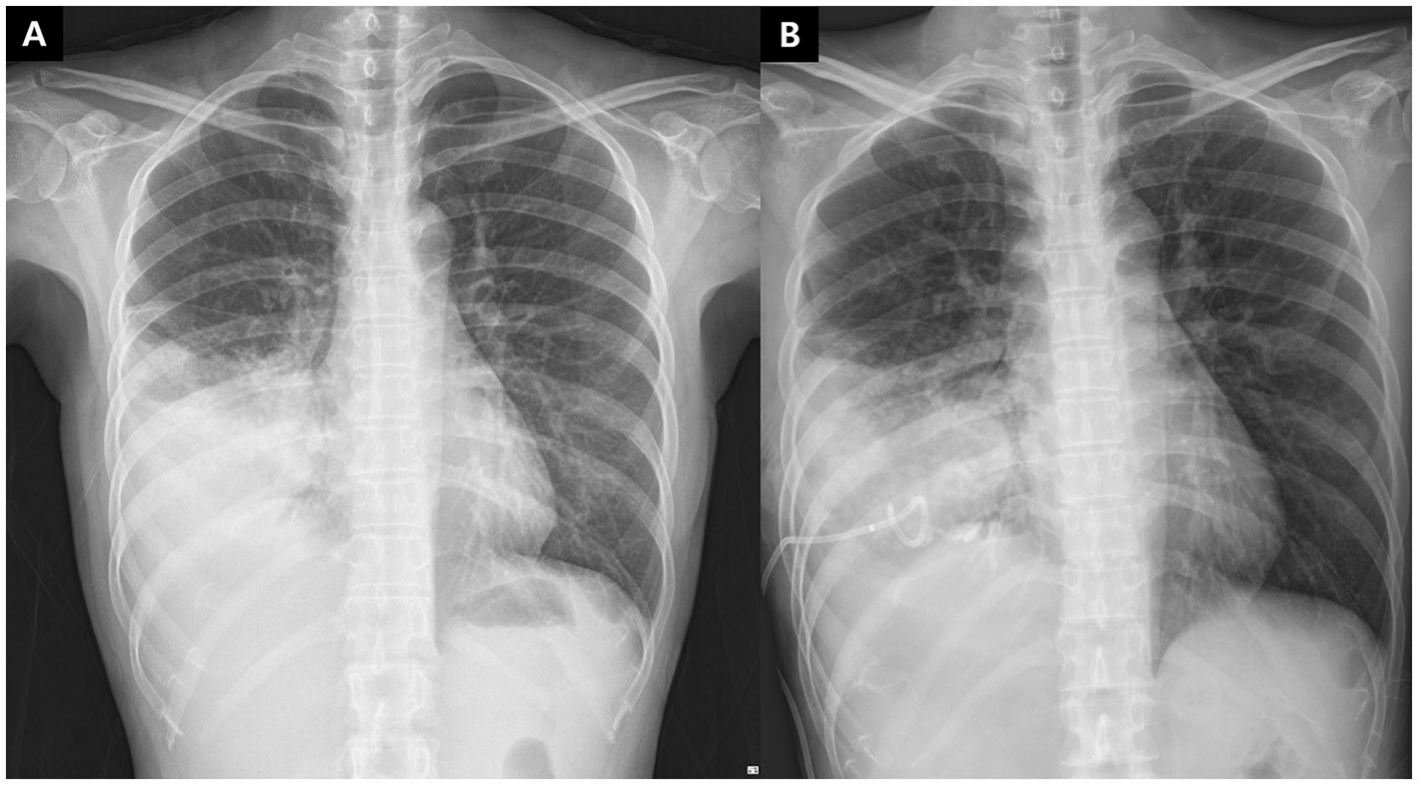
Chest radiographs of the patient. (A) Initial chest X-ray at admission. (B) Chest x-ray 3 days after the initial visit, following percutaneous catheter drainage insertion.
